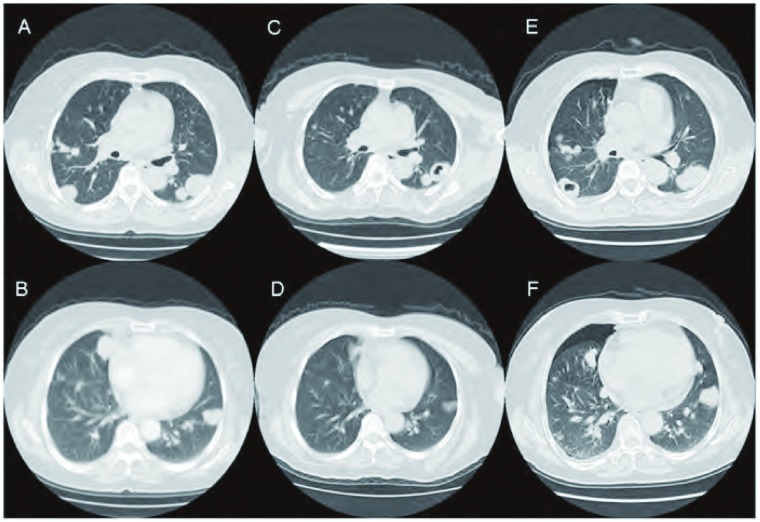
患者治疗前后的胸部CT表现。A、B：基线检查显示双肺多发结节影（2007-12-10）；C、D：6周期贝伐珠单抗合用化疗后显示双肺病变明显缩小及空洞改变（2008-05-19）；E、F：贝伐珠单抗维持治疗39个月时CT显示双肺病变增多增大，病情进展且右肺出现自发性气胸（2011-03-04）。 The computed tomography scans before and after treatment. A, B: The CT scans (10-Dec-2007) of the baseline showed multiple massive and nodular shadows in both lungs; C, D: The CT scans (19-May-2008) after six cycles of chemotherapy and bevacizumab showed that the tumor began shrinking obviously with cavity formation; E, F: The CT scans of the maintenance therapy with bevacizumab for 39 monthes (04-Mar-2011). The tumor sizes had been increasing, pneumothorax in the right lung can be seen and new metastatic nodules were also found in the lungs.
